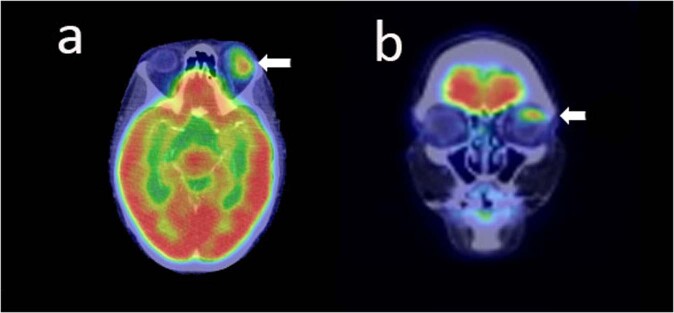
PET imaging in MALT. a Axial and b Coronal, ^18^F-FDG PET scan showing avidity at the left superior fornix, later confirmed to be EMZL.
